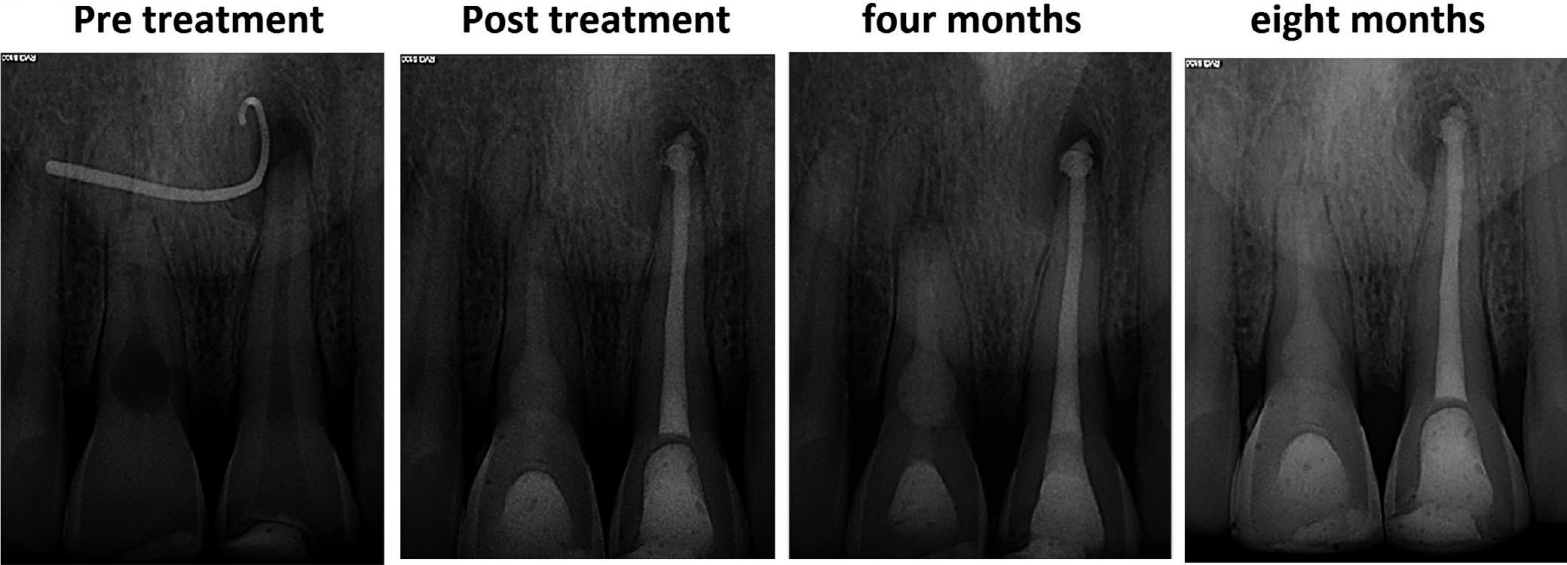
Pretreatment, post‐treatment, 3‐month, and 8‐month follow‐up radiographs
